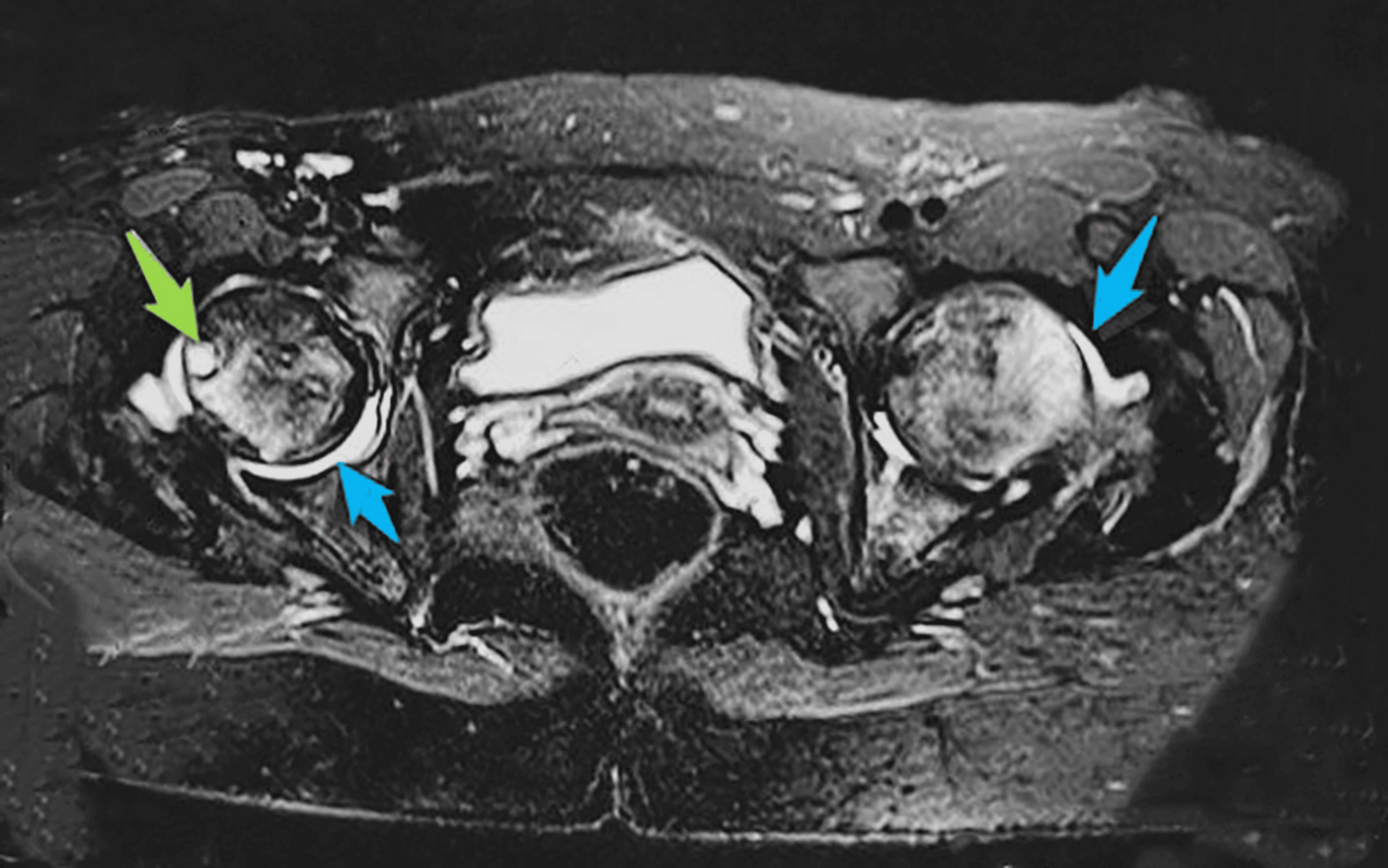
Bilateral hip MRI indicating joint effusion (blue arrow) and a subchondral cyst on the right side (green arrow)
